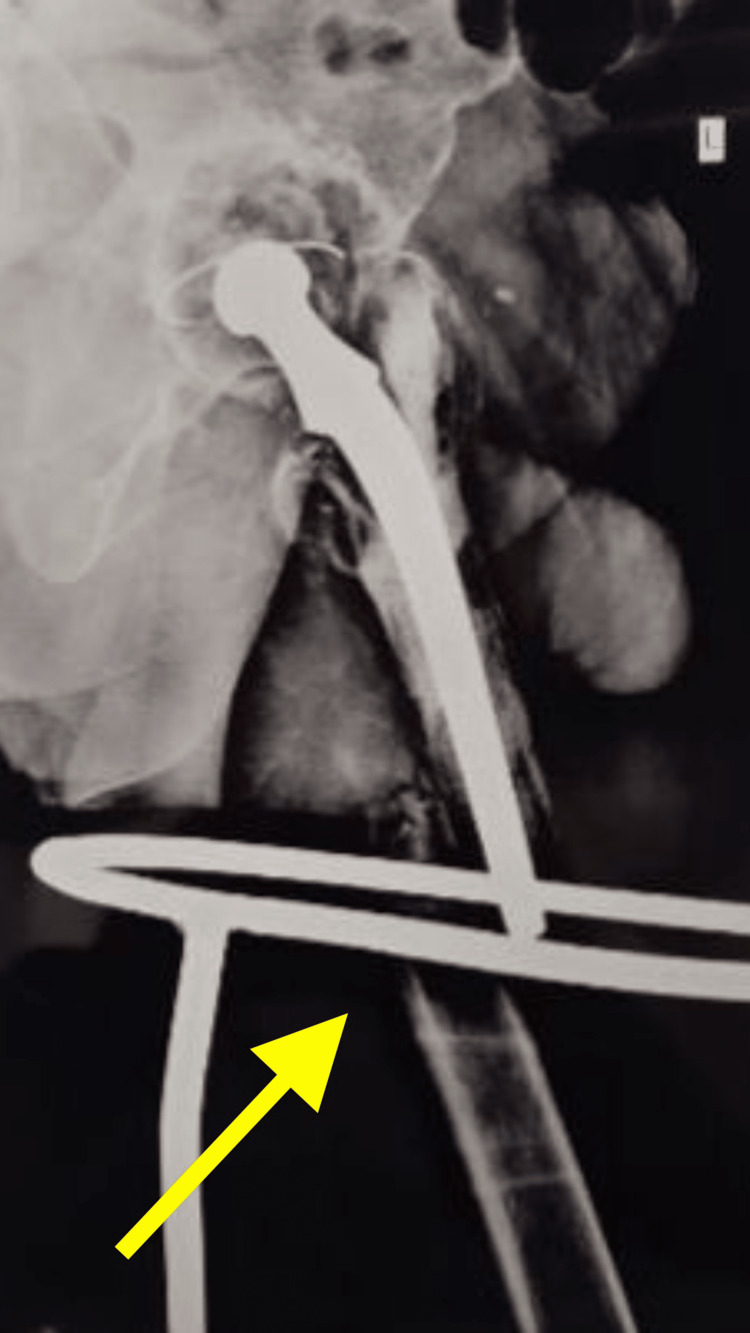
Pre-operative radiograph of the left hip with thigh lateral view showing periprosthetic femur fracture left side
